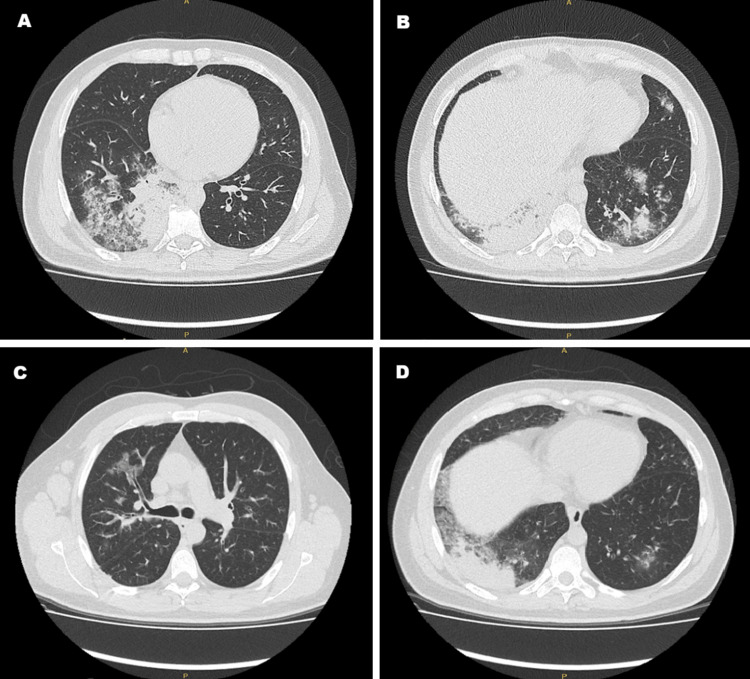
High-resolution computed tomography (HRCT) of the chest during the first (A and B) and second episode (C and D) A and B: An alveolar occupation pattern with consolidative appearance is observed, associated with air bronchogram configuration and peripheral ground-glass opacities at the level of the posterobasal segments of both hemithoraces, with greater involvement of the right lung. C and D: Multiple areas of ground-glass opacities are identified in the right upper lobe and right lower lobe. A posterior basal right parenchymal consolidation with peripheral ground-glass opacities and air bronchogram is noted. Incipient ground-glass areas are also seen in the left lung.
